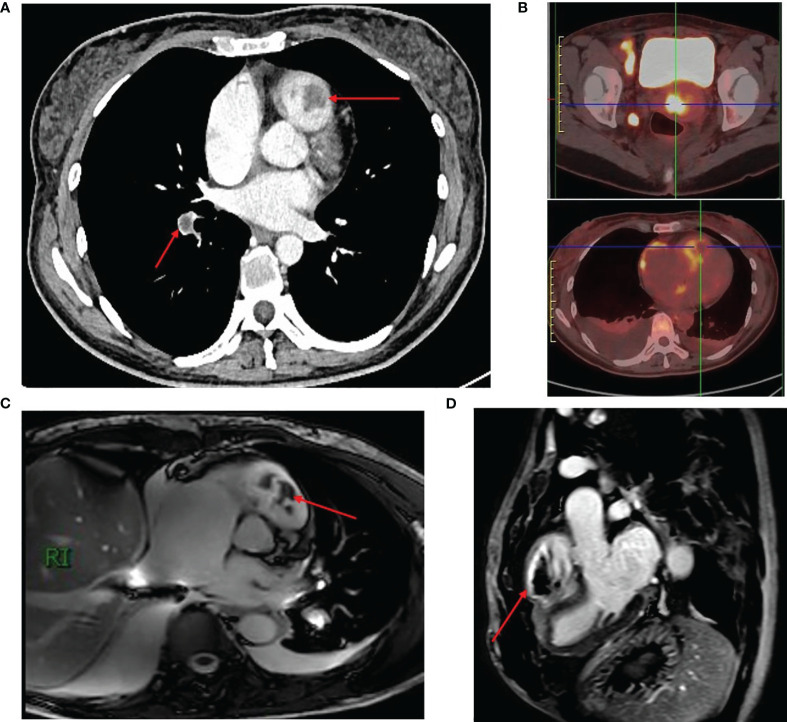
Radiological Findings in a Case of Advanced Cervical Cancer with Right Ventricular Thrombosis and Metastasis. (A) Enhanced CT scan showing right ventricular thrombosis and pulmonary embolism. (B) PET-CT scan revealed a cervical mass, lymph node enlargement, and tumor thrombus in the right ventricle. (C, D) Cardiac MRI demonstrating thrombosis with right ventricular. The red arrows represent right ventricular and pulmonary thrombus.
